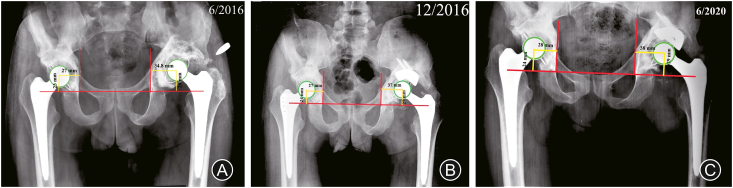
Follow-up radiographs. (A) Loosening and proximal migration of the left acetabular component; (B) Revision of the acetabular component by a cemented cup and a metal augment; (C) Septic loosening of the left acetabular cup.
